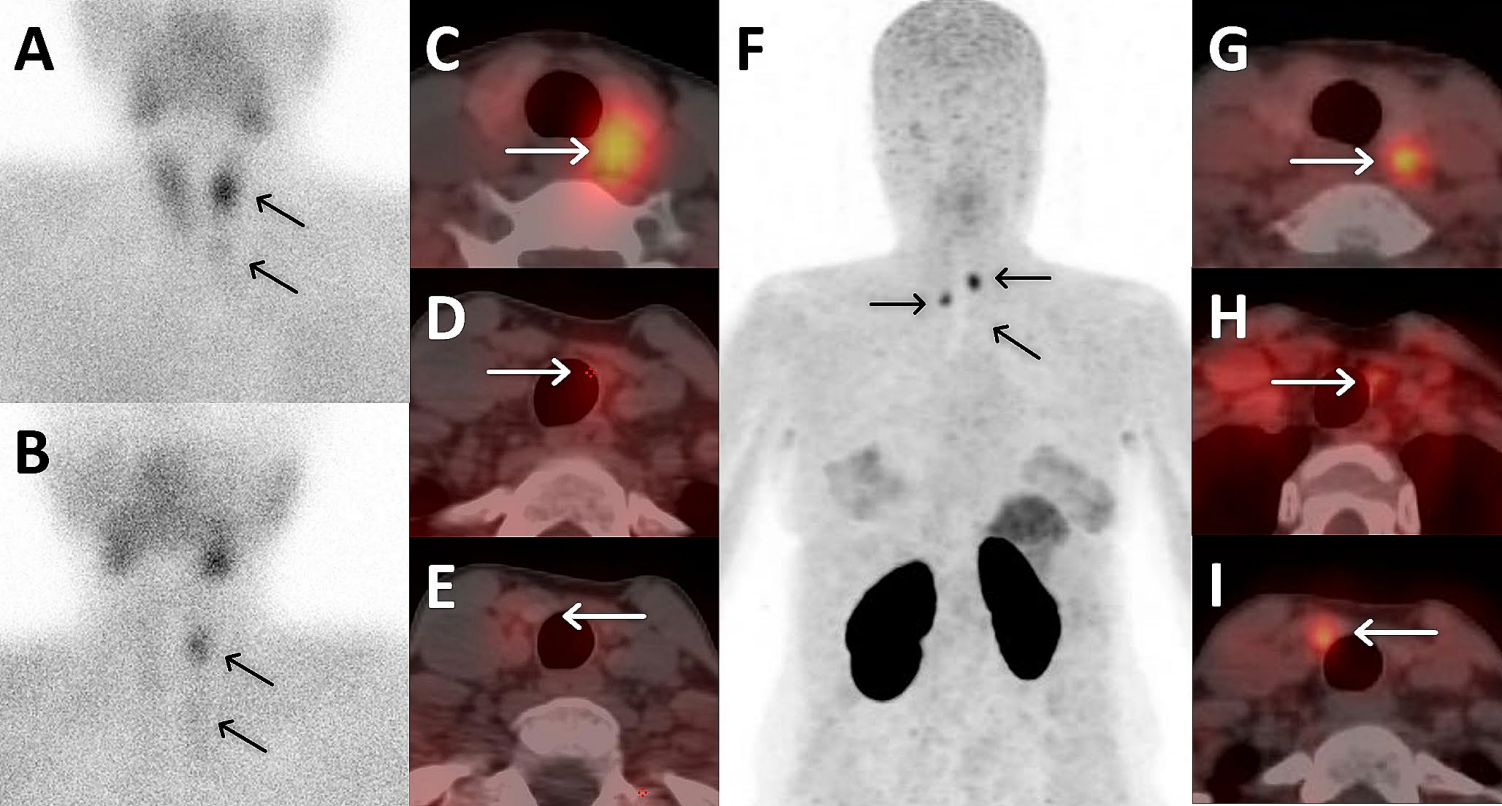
A 34-year-old woman diagnosed with MEN-1 syndrome and PHPT (PTH: 122 ng/l, Calcium: 10.6 mg/dL) underwent MIBI scan, showing intense activity in a lesion located posteriorly to the left lobe of the thyroid gland (A, B, C), and mild uptake in an inferiorly located lesion (D); yet no uptake was observed in a lesion inferior to the right lobe (E). Additional ^68^Ga-Trivehexin PET/CT uptake confirmed parathyroid pathology in all three lesions (F, G, H, I) through PTH wash-out
